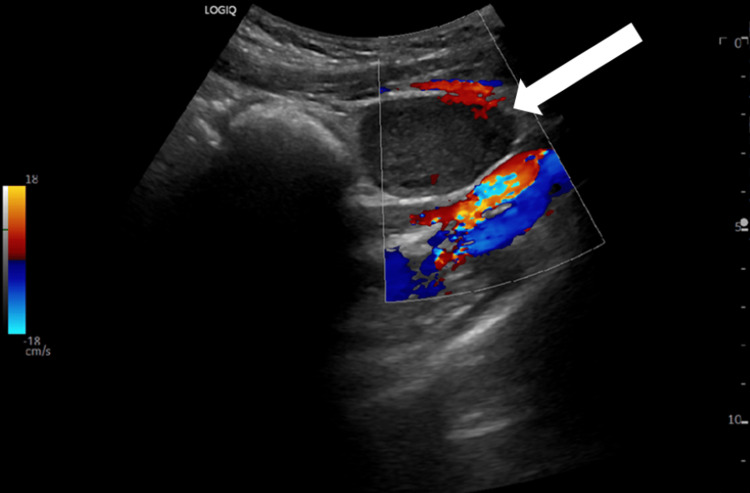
Transverse view of the left ovary with Doppler imaging.
